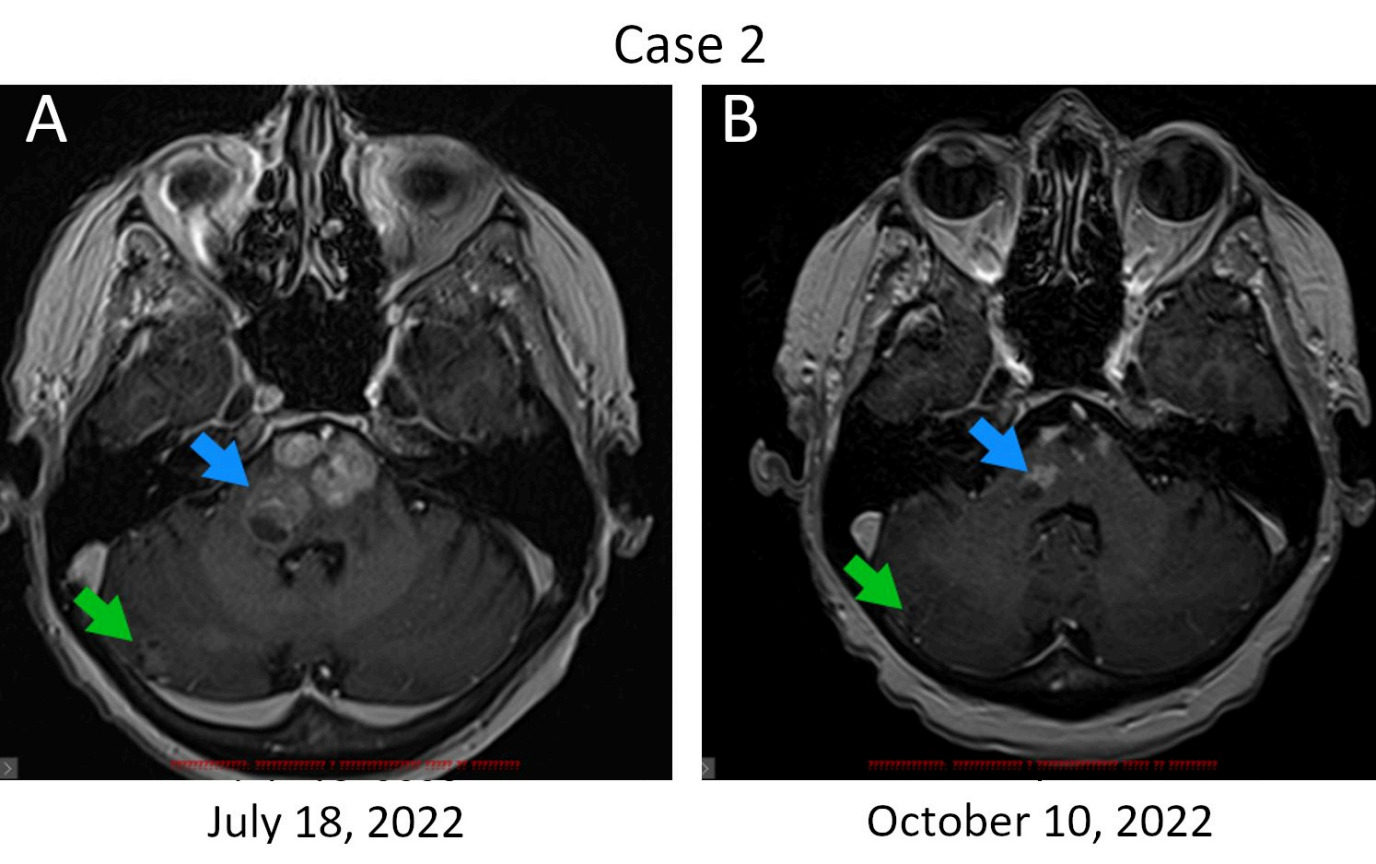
Regression of brain metastases in response to bevacizumab therapy. (A) Metastatic lesions in the brain at baseline. Bevacizumab infusions were started on September 16, 2023; (B) MRI examination was carried out on day 24 after the first dose of this drug. The reduction of the size of metastatic lumps is observed in the pons Varolii (from 19 mm × 15 mm to 13 mm × 8 mm and from 14 mm × 8 mm to 8 mm × 5 mm, blue arrow) and in the right hemisphere of the cerebellum (from 11 mm × 10 mm to 9 mm × 6 mm, green arrow). The sum of diameters of target lesions has been reduced by 32%
